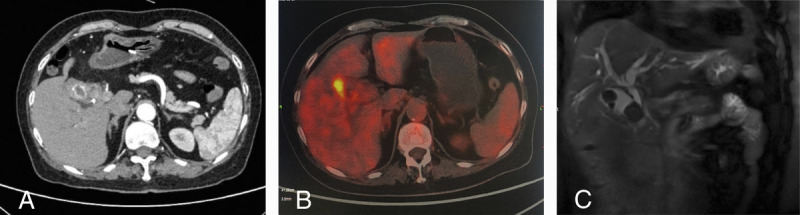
A, Contrast-enhanced CT images revealed gallstones with thickening and strengthening of the gallbladder wall. B, PET/CT detected high-density shadows in the gallbladder, thickened wall of the gallbladder and common hepatic duct (involving adjacent hepatic parenchyma), and a focal hypermetabolic lesion (SUV_max_, 6.1) in and around the area. C, MRCP revealed multiple gallstones and extrinsic compression of the extrahepatic biliary tract, all indicative of Mirizzi syndrome. CT: Computed tomography; MRCP: Magnetic resonance cholangiopancreatography; PET: Positron emission tomography; SUV_max_, Maximum standard uptake value.
